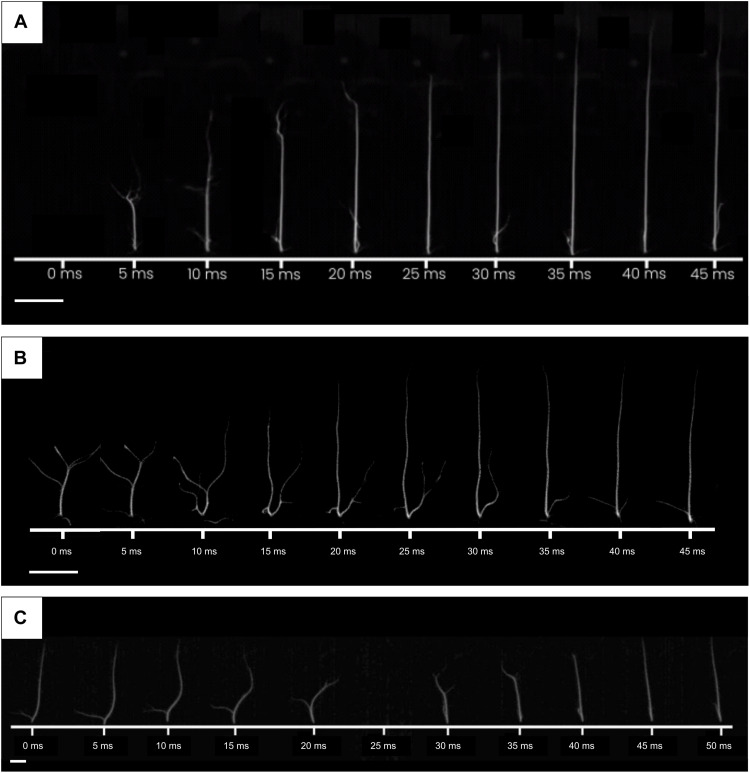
Spark being guided at different time stamps. (A) The spark is switched on when the ultrasonic field is already on. (B) The spark is already on, and the ultrasonic field is switched on at *t* = 0. (C) The spark is tilted right to left with dynamic array control. Scale bars, 1 cm.
